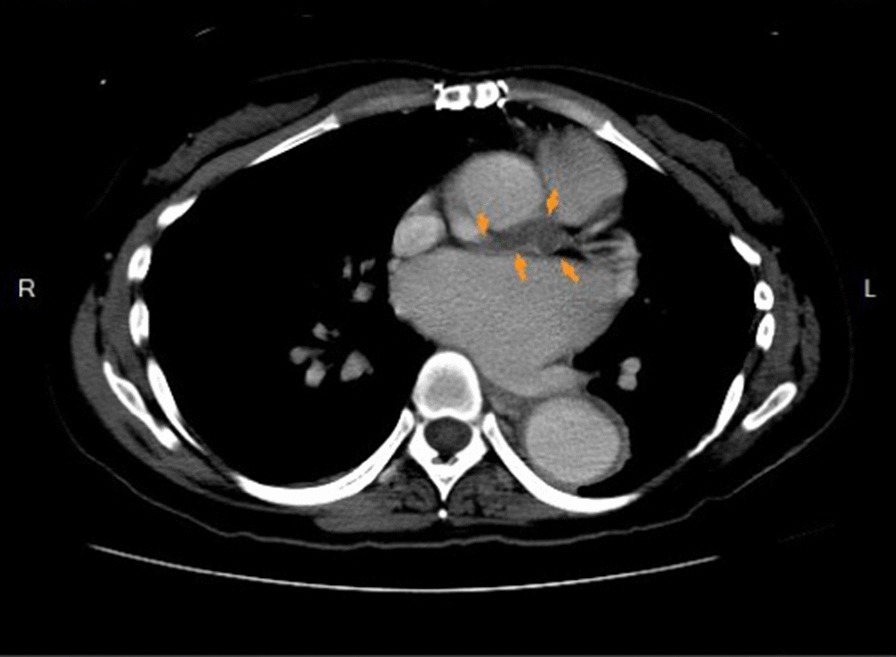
Contrast CT showed a thrombosis in the Dacron graft (Ar-row)
